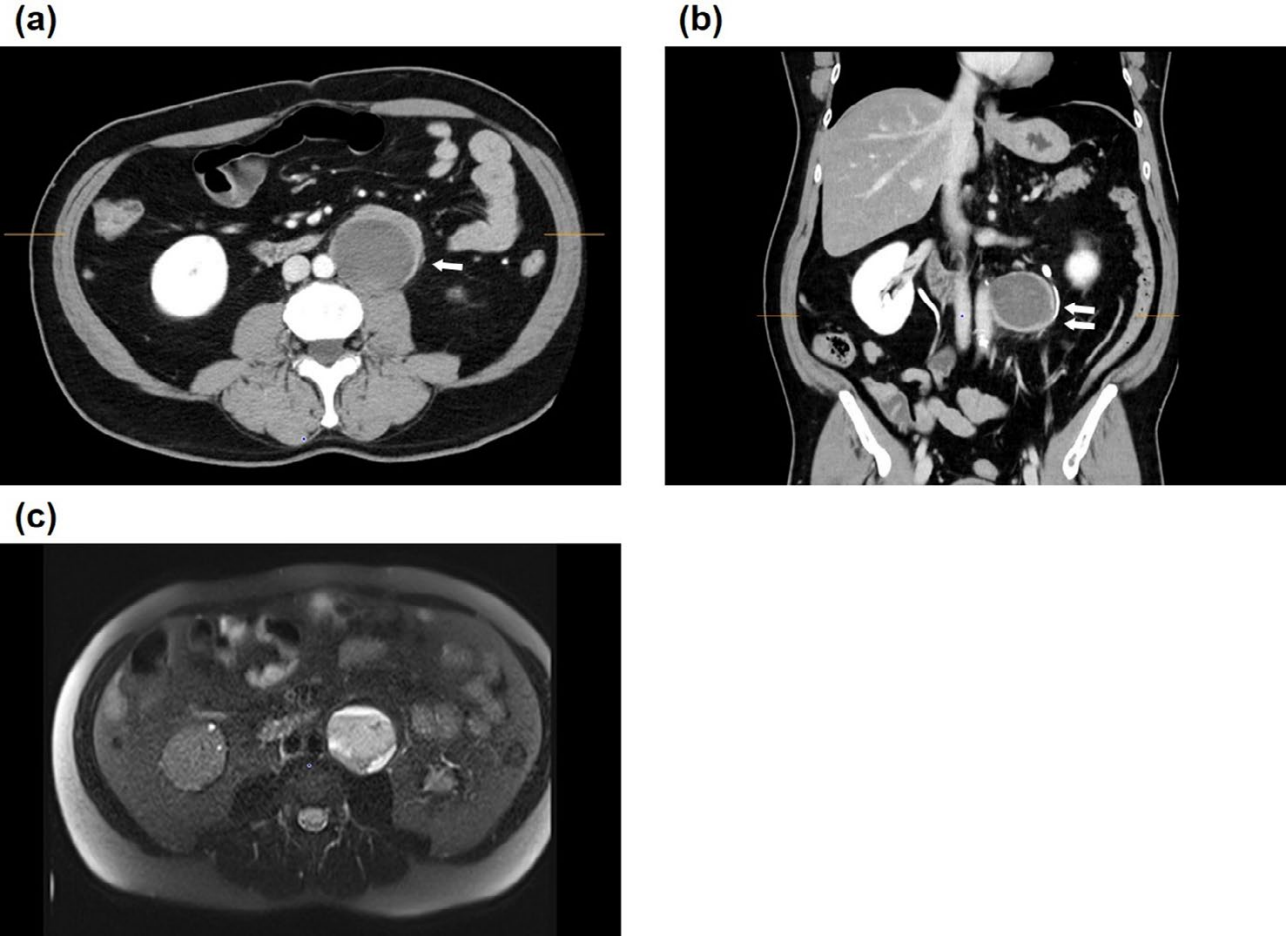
Abdominal CT and MRI findings. (a) Contrast‐enhanced CT scan showing a cystic mass in the left retroperitoneum, adherent to the abdominal aorta. The tumor measured 6.5 cm in size. A peripheral solid component of the cystic mass demonstrated progressive contrast enhancement. The left ureter (arrows) was adherent to the tumor (axial view). (b) On the excretory phase of contrast‐enhanced CT, the left ureter (arrows) was observed to be adherent to the tumor (coronal view). (c) On T2‐weighted MRI, the lesion exhibited high signal intensity, with a cystic component. CT, computed tomography; MRI, magnetic resonance imaging.
